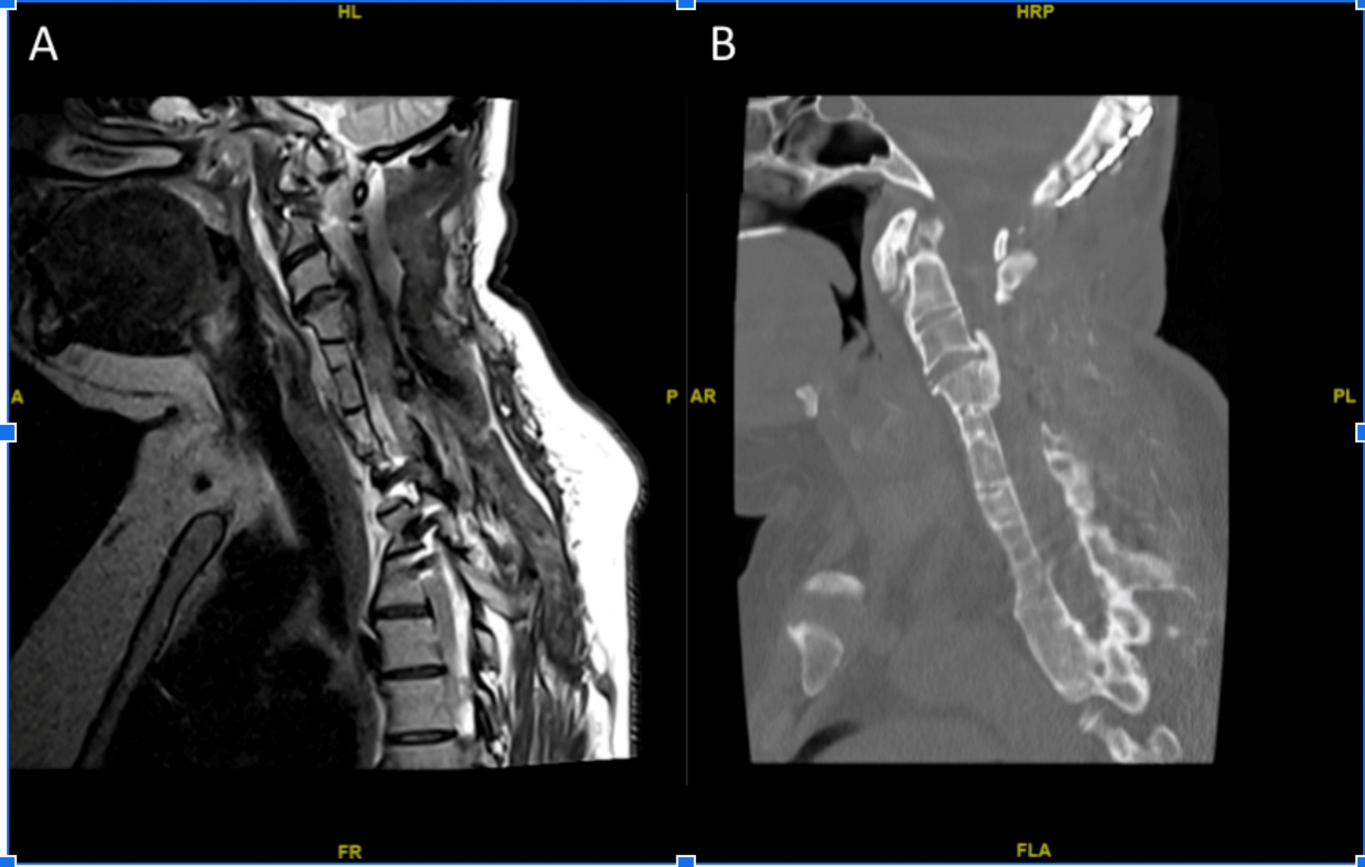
(A) Sagittal T2-weighted MRI view showing severe ventral spinal cord compression at the level of C3. (B) Sagittal CT scan demonstrating congenital segmentation anomalies consistent with Klippel-Feil syndrome, including fusion of cervical vertebrae, hypoplasia of the clivus, and a cranially positioned odontoid process.
